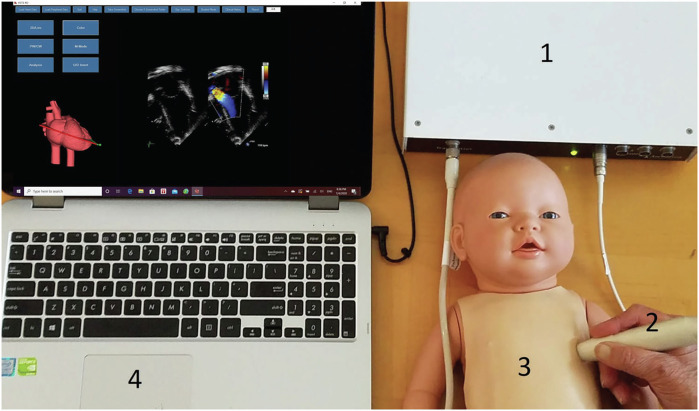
Virtual Neonatal Echocardiography Training System (VNETS): figure showing the VNETS system in use with tracking system device, mannikin, and computer.
